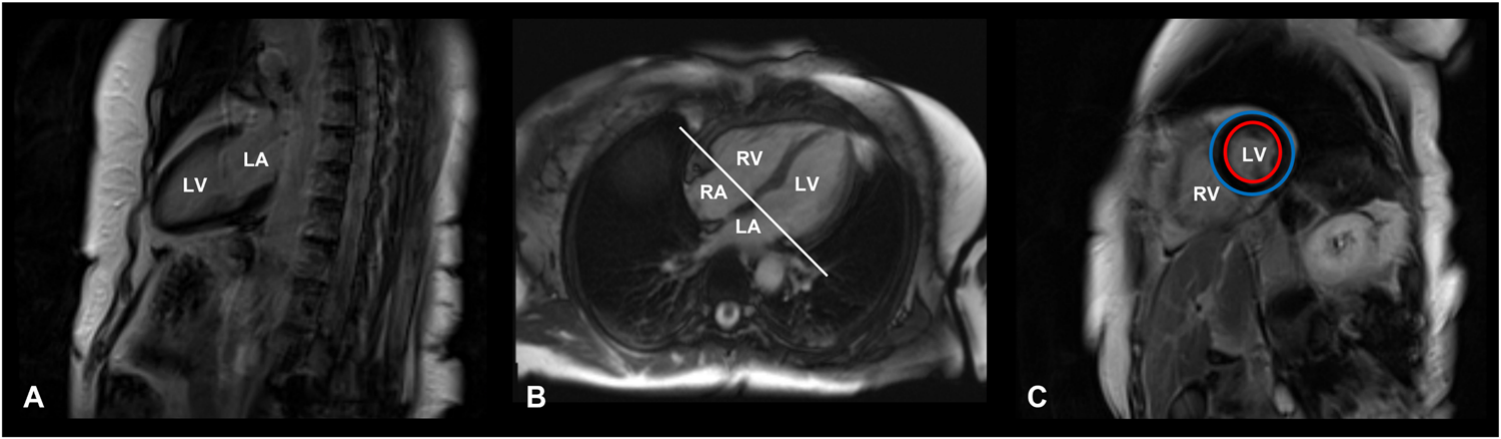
Cardiac magnetic resonance image acquisition protocol. T1-weighted, late gadolinium enhancement cardiac MR images of a 71-year-old female patient with Cushing’s disease, cured after successful neurosurgery. A Vertical long axis slice, coronal plane, two-chamber view. B Horizontal long axis slice, axial plane, four-chamber view. C Short-axis slice at the end of the diastole, sagittal plane. Red circle: endocardium; Blue circle: epicardium. LA left atrium, LV left ventricle, RA right atrium, RV right ventricle
